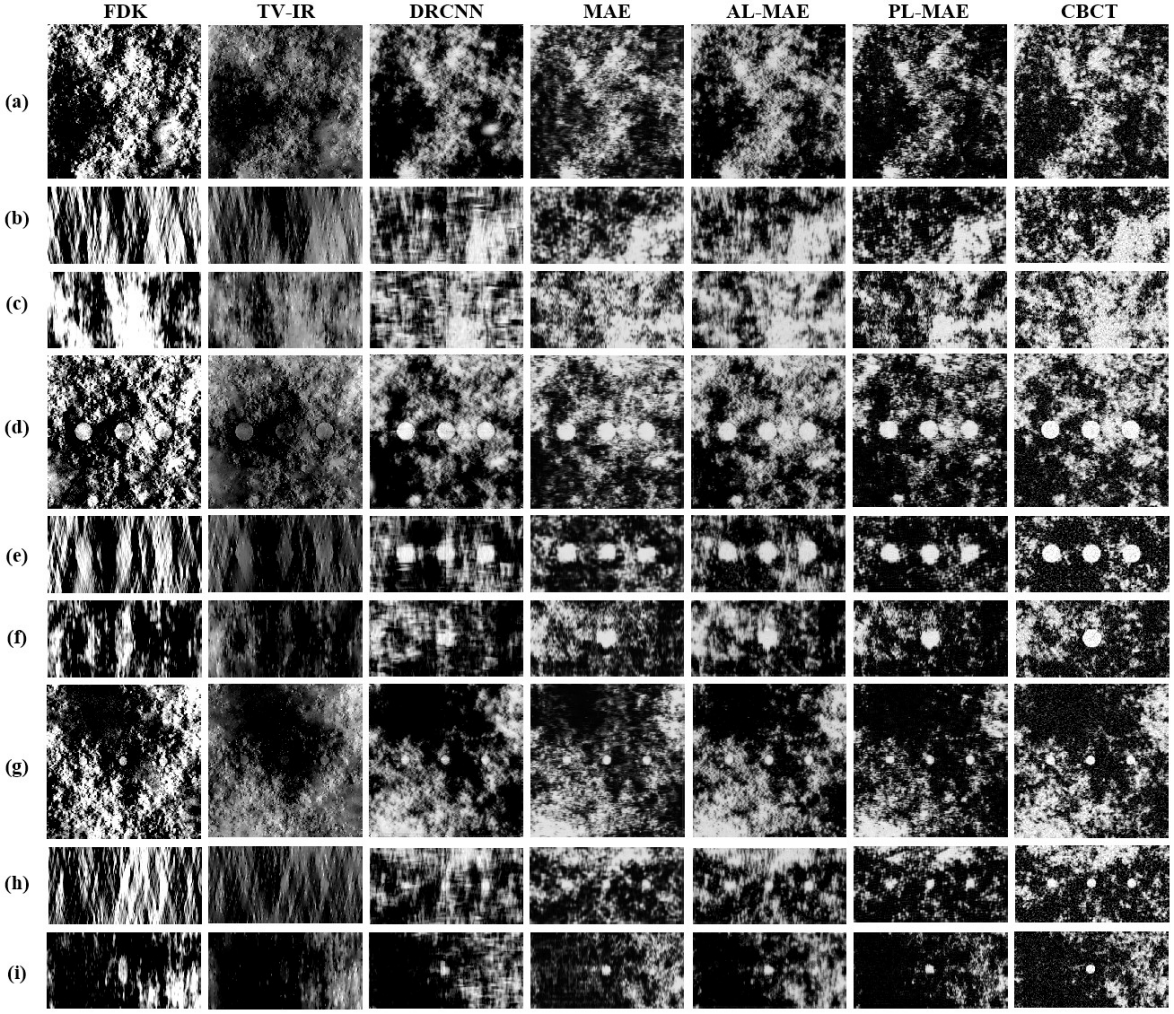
Results using 30% VGF DBT images. DBT images reconstructed with FDK and TV-IR, deblurred images by DRCNN, deblurred images by the proposed method with MAE, AL-MAE, and PL-MAE loss functions, and CBCT images (from left to right). Images without mass lesion for (a) axial, (b) coronal, and (c) sagittal planes; images containing 4 *mm* lesions for (d) axial, (e) coronal, and (f) sagittal planes, and images containing 2 *mm* lesions for (g) axial, (h) coronal, and (i) sagittal planes. The display window is [0.0456 0.0844] in *cm*^−1^.
